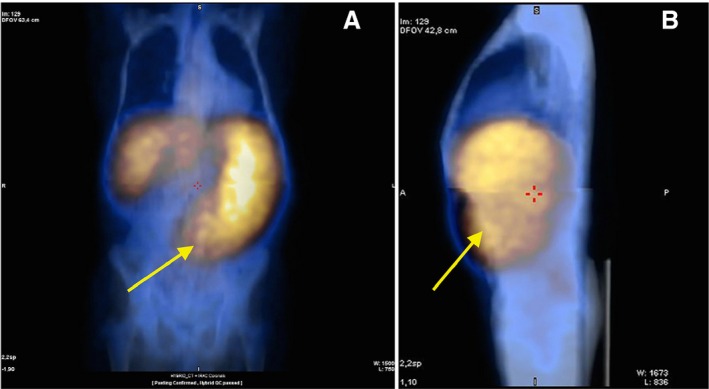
^111^In‐Cl_3_ single‐photon emission tomography/computed tomography imaging in one patient with very advanced primary myelofibrosis: (A) front view and (B) side view showing very intense uptake of the radiotracer in the spleen (yellow arrow) and almost no fixation in the backbone and other structures of the axial skeleton.
